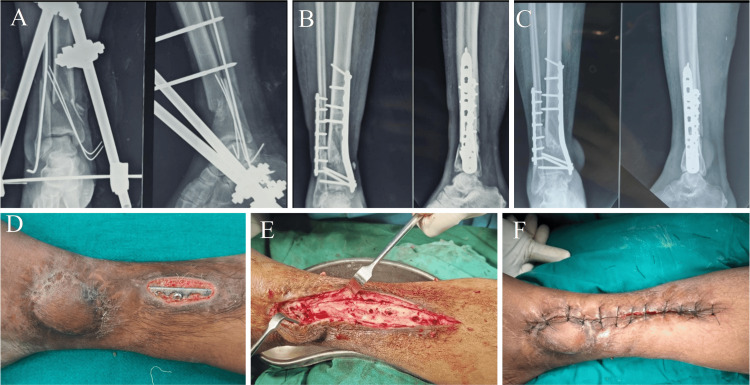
Case 2 dual-incision approach (A) A 40-year-old female with a history of RTA resulting in an open distal tibial and fibular fracture, Grade 3b. The compound wound was on the medial side and was managed with external fixation. (B) After plastic surgery intervention, the patient was treated with MIPO tibia and ORIF fibula. (C) X-ray after 11 months of follow-up. (D) Follow-up showed wound dehiscence and implant exposure on the medial side. (E, F) Implant removal was performed after 11 months. RTA: road traffic accident, MIPO: minimally invasive plate osteosynthesis, ORIF: open reduction and internal fixation
